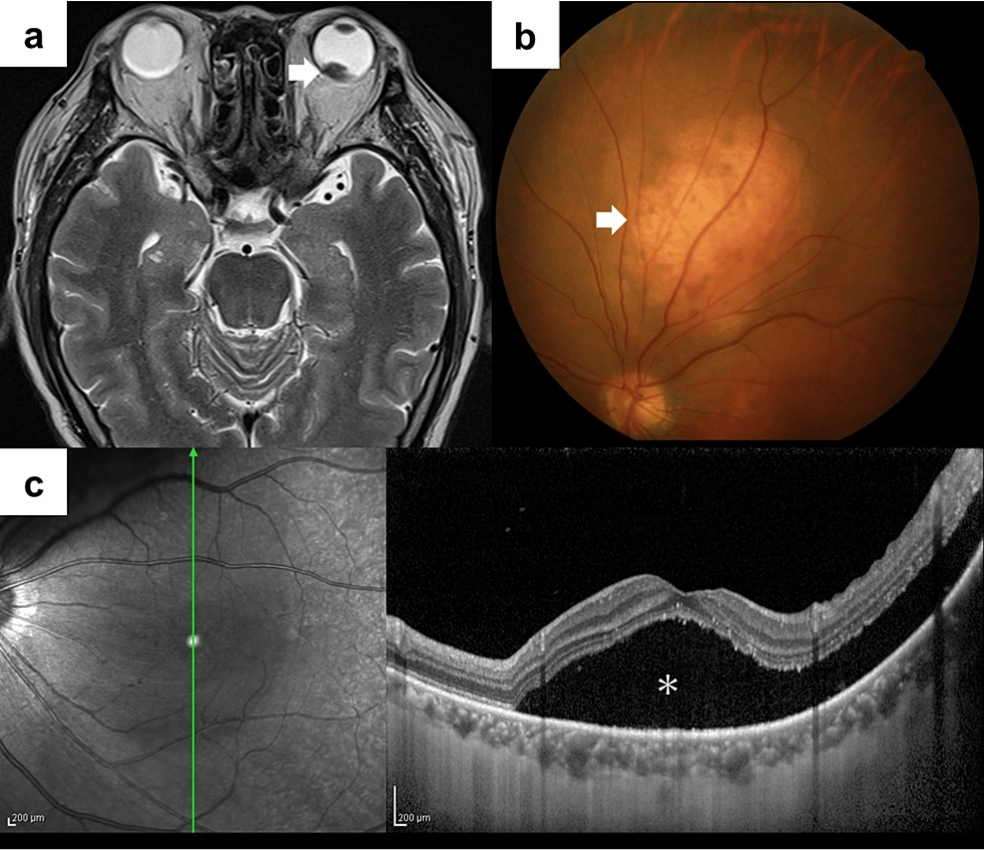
Magnetic resonance imaging, fundoscopy, and optical coherence tomography of the choroidal metastasis a: Magnetic resonance imaging (T2-weighted imaging) revealing an intraocular tumor in the left eye (arrow). b: Fundoscopy demonstrates pigmentary changes suggesting a neoplastic lesion (arrow). c: Optical coherence tomography at the level of the green arrow shows subretinal fluid and serous retinal detachment (*).
